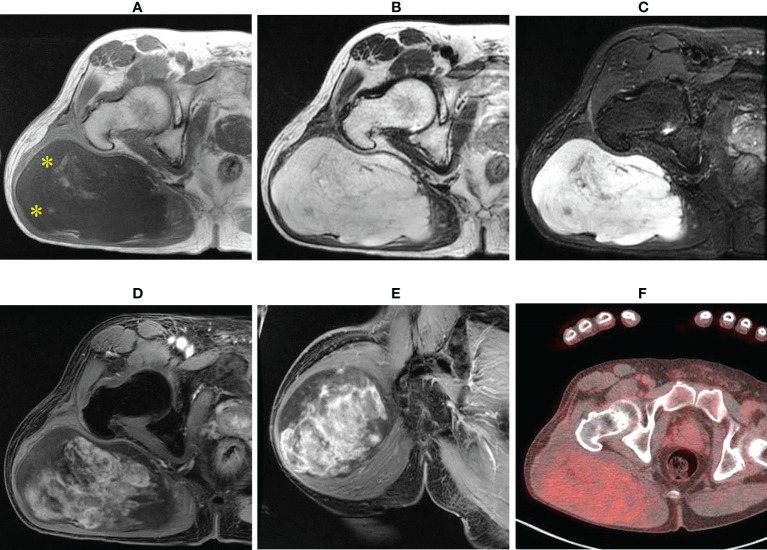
Imaging findings. T1-weighted axial images showing low and slightly high signals (yellow asterisk) (A); almost homogeneous high signal on T2-weighted images (B) and short tau inversion recovery images (C); and axial and central enhancement on a gadolinium-enhanced T1-weighted axial image (D, E). Axial fluorodeoxyglucose (FDG) positron emission tomography-computed tomography (PET-CT) showing a hypometabolic tumor (F).
